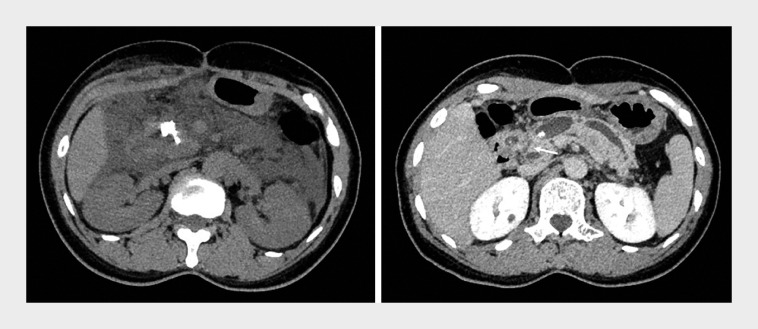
Computed tomography (CT) showed extensive exudation and peripancreatic fluid collection, and impacted stones in the pancreatic head (left). Enhanced CT revealed a suspected connection (arrow) between the common bile duct and the main pancreatic duct (right).
